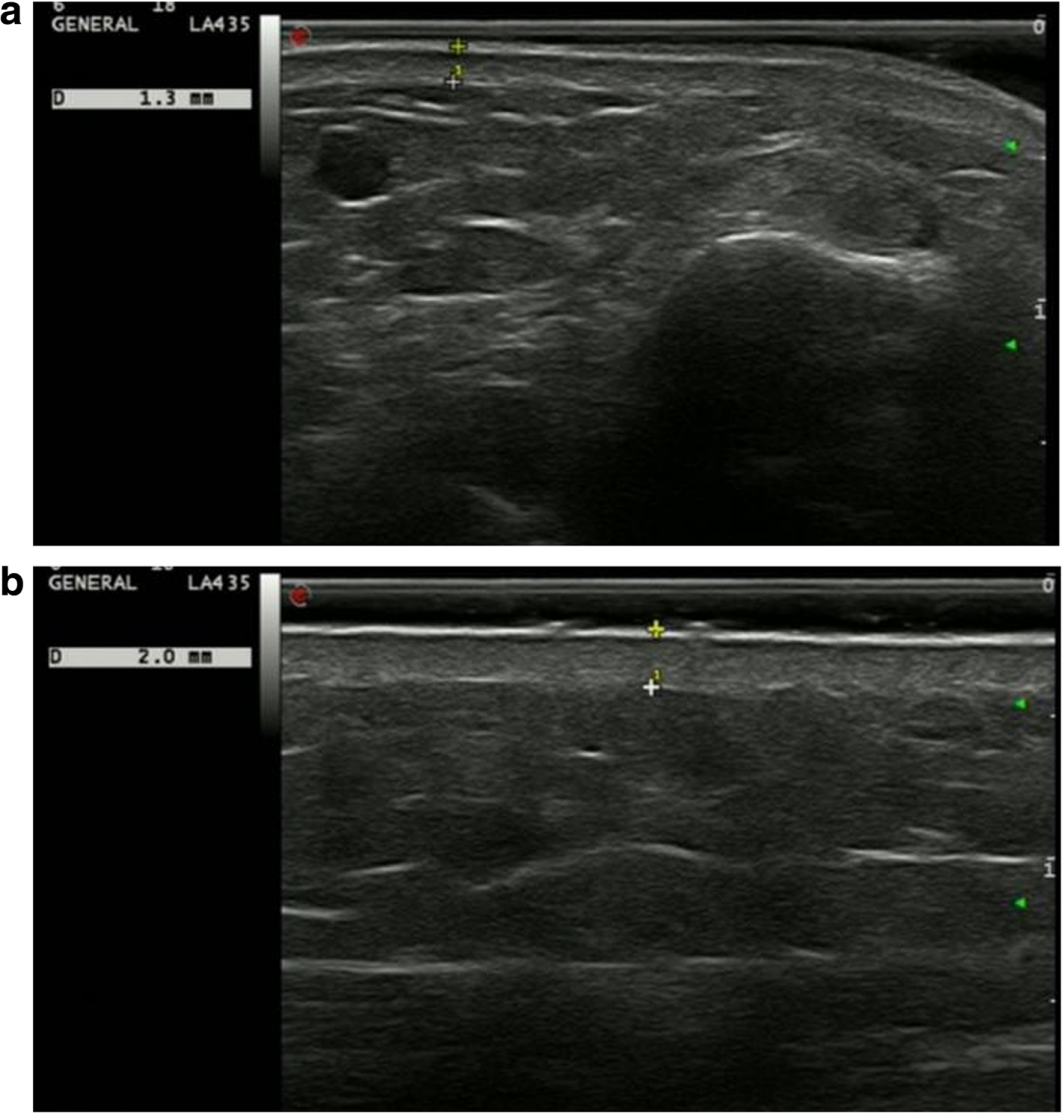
High-frequency ultrasound, 18-MHz echograms (vertical images) highlight the epidermis and dermis at the forearm in a healthy control and a patient with systemic sclerosis. The skin thickness was 1.3 mm (between the plus sign) in the control (a), and 2.0 mm (between the plus sign) in the patient (b)
